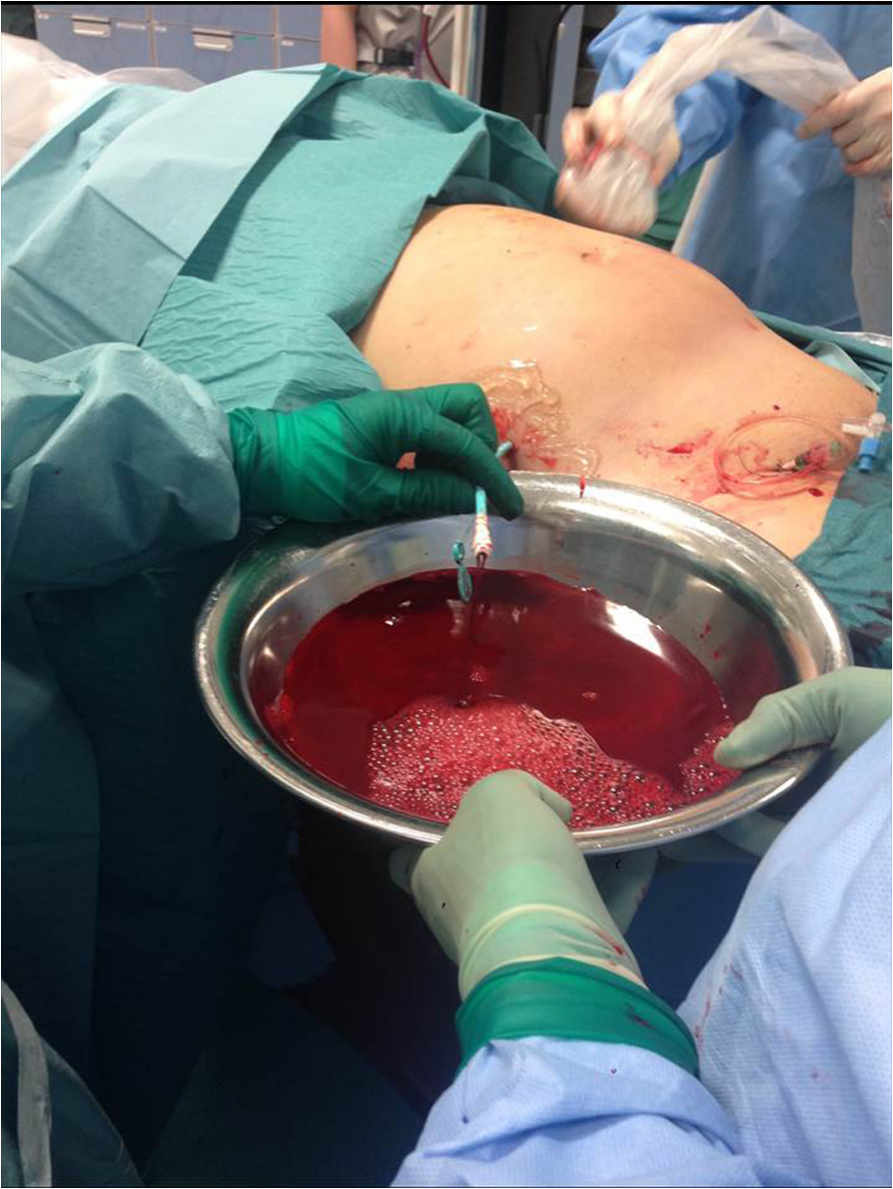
Rapid evacuation of massive hemoperitoneum through a 16-French pigtail catheter inserted under ultrasound guidance
